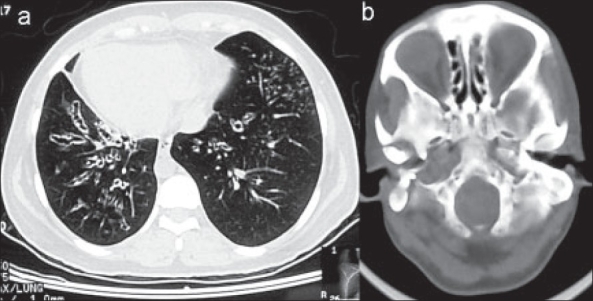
a) HRCT thorax. b) Axial CT PNS [bone window]
